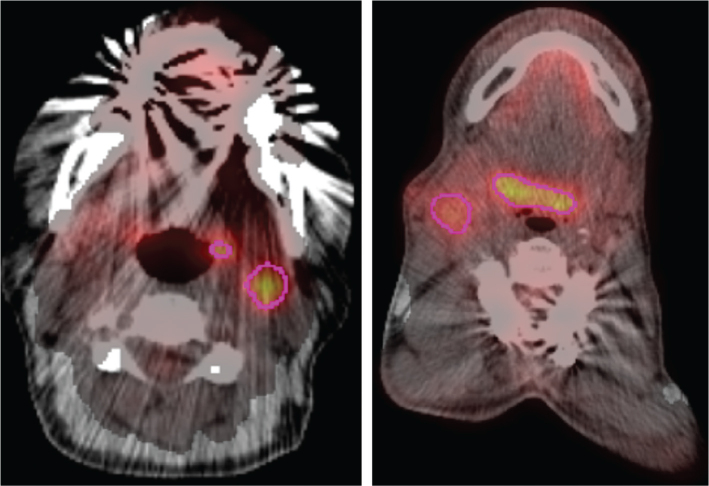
AI-PET-GTV output in two cases of unknown primary head and neck cancer. Left: A patient initially managed with surgical resection of a left-sided lymph node, later diagnosed with oropharyngeal cancer. The AI-PET-GTV correctly highlighted the oropharynx as the primary site on the pre-treatment PET-CT scan. Right: A patient with a right-sided nodal presentation who underwent nodal dissection before diagnosis of an oropharyngeal primary; here too, the AI-PET-GTV delineation correctly localised the oropharynx on the diagnostic scan. In both cases, the AI model identified the primary site before clinical diagnosis, supporting its potential value in unknown primary tumour localisation.
